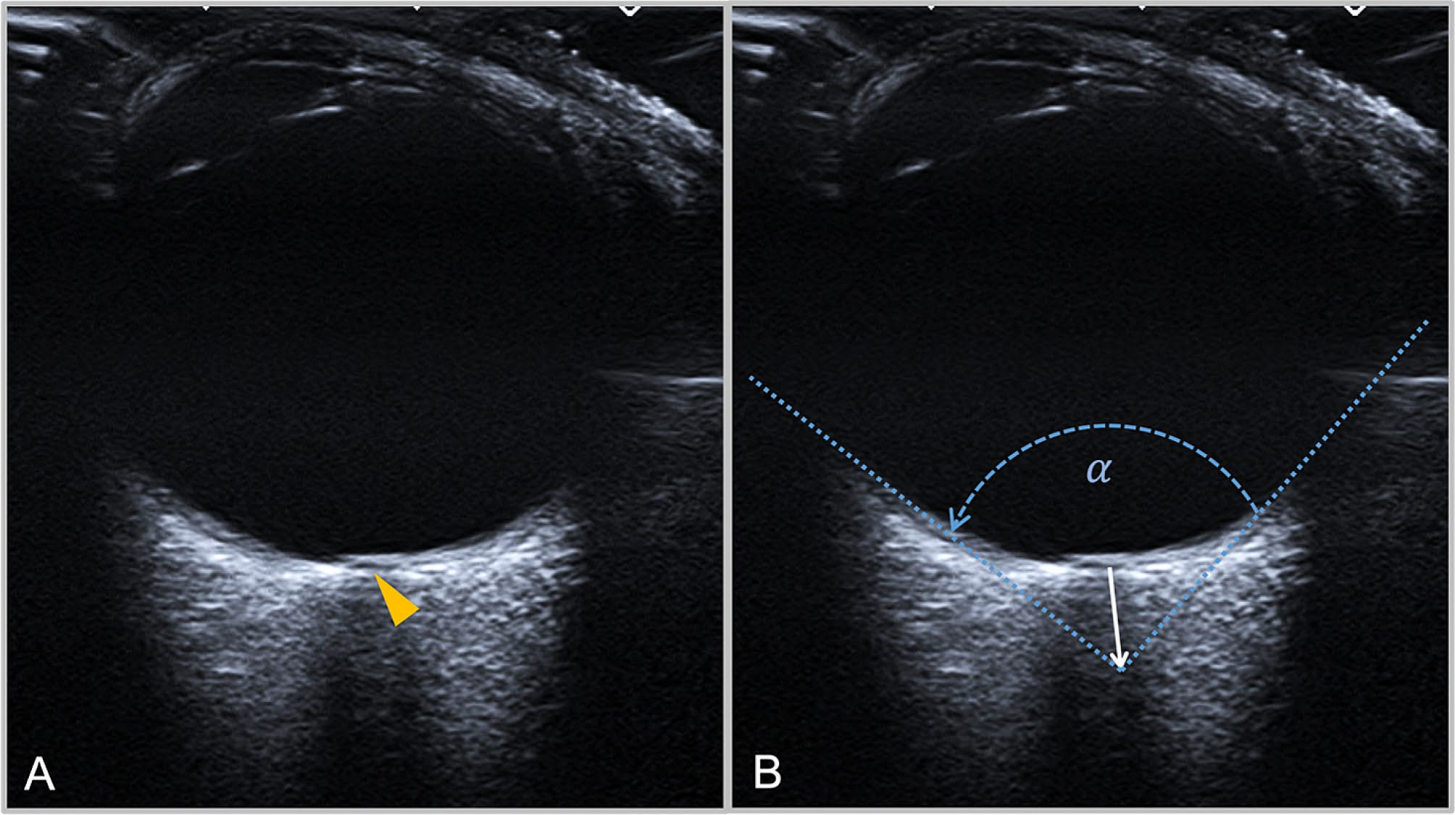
Transorbital ultrasound of one globe in the axial plane. Picture A shows the original image, the yellow arrowhead marks the ophthalmic lamina cribrosa. Picture B shows exemplary measurement of the PGA at 3.0 mm distance from the ophthalmic lamina cribrosa
